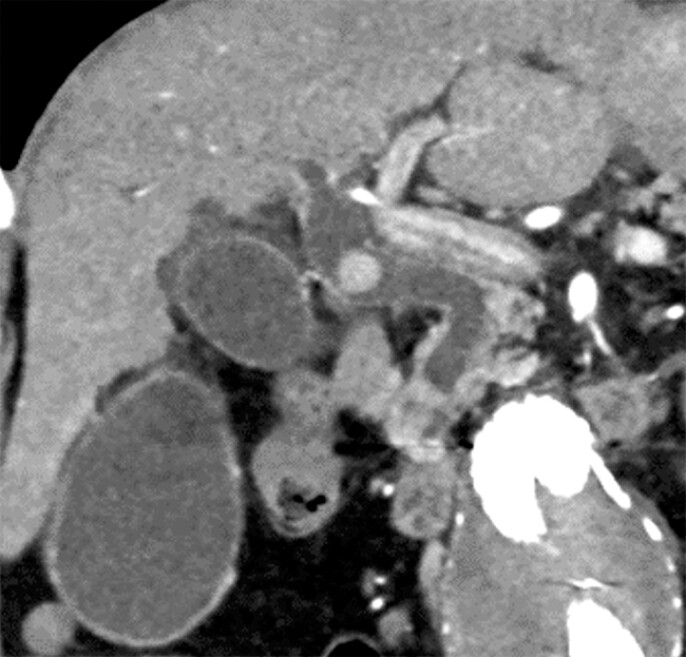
Computed tomographic image showing a 15-mm stone in a highly angulated common bile duct.
